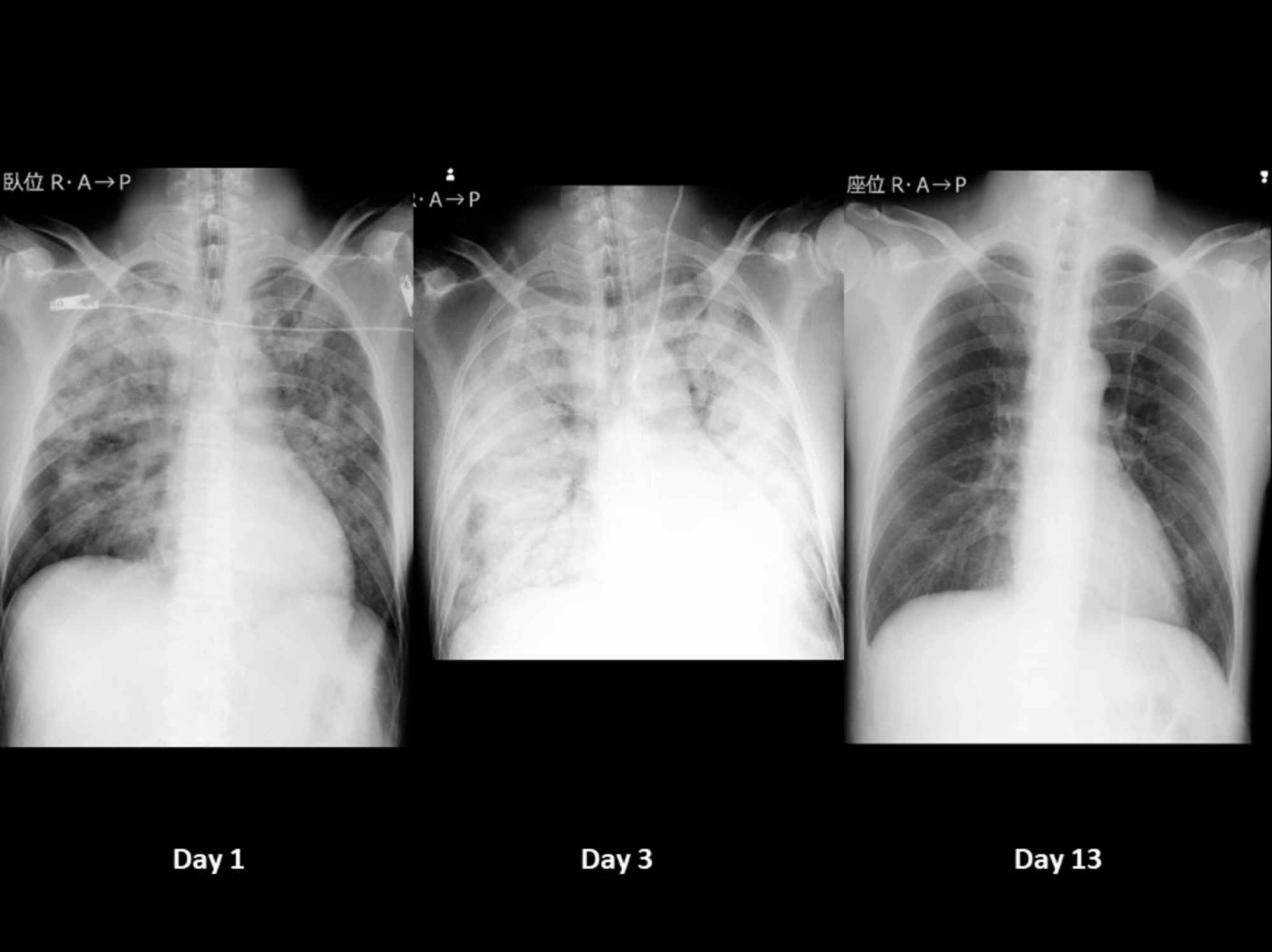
Time course of chest X-ray The patient was managed by extracorporeal membrane oxygenation and mechanical ventilation with the lung rest setting on the third day and was withdrawn from mechanical ventilation on the 13th day Day 1 (left) shows bilateral ground-glass appearances. Day 3 (middle) shows the deterioration of bilateral radiolucency. Day 13 (right) shows clear lung fields
